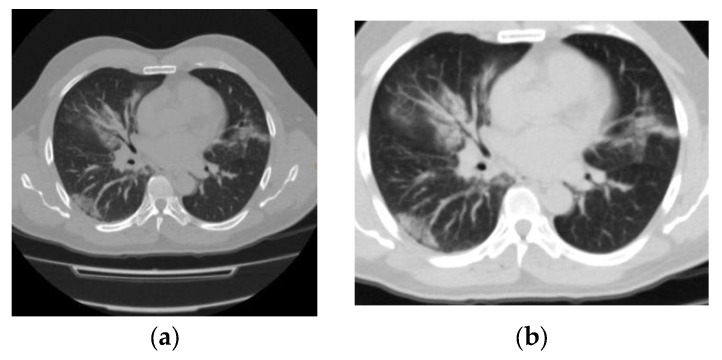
(a) Axial section and (b) axial section (with magnification) show a pattern of multifocal interstitial ground-glass opacity characteristic of pneumonia associated with COVID-19 (SARS-CoV-2) and highly suggestive of the disease in this patient. She was admitted to the intensive care unit after chest CT, showed a positive RT–PCR test result 48 h later, and died 7 days after admission.
